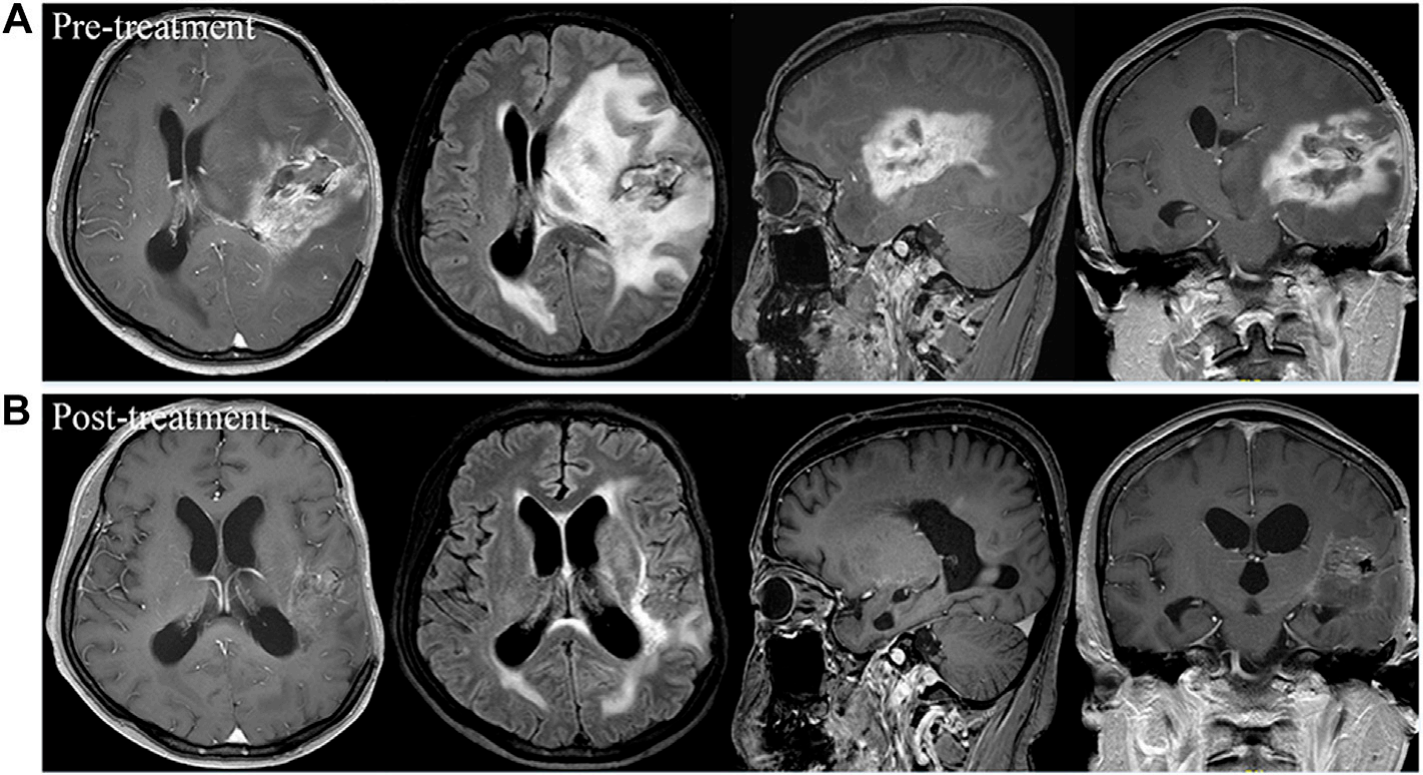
Brain scan of a patient with left frontotemporal glioblastoma multiforme who had relapsed from surgery, standard chemoradiotherapy regimen, and ten cycles of adjuvant temozolomide, and then had progressed after bevacizumab treatment. Comparison of imaging findings between pre-treatment (A) and post-treatment at 4 months of apatinib monotherapy (B) by contrast-enhanced MRI and MRI-Flair. The patient achieved partial response after treatment and had a progression-free survival time of 5.3 months.
